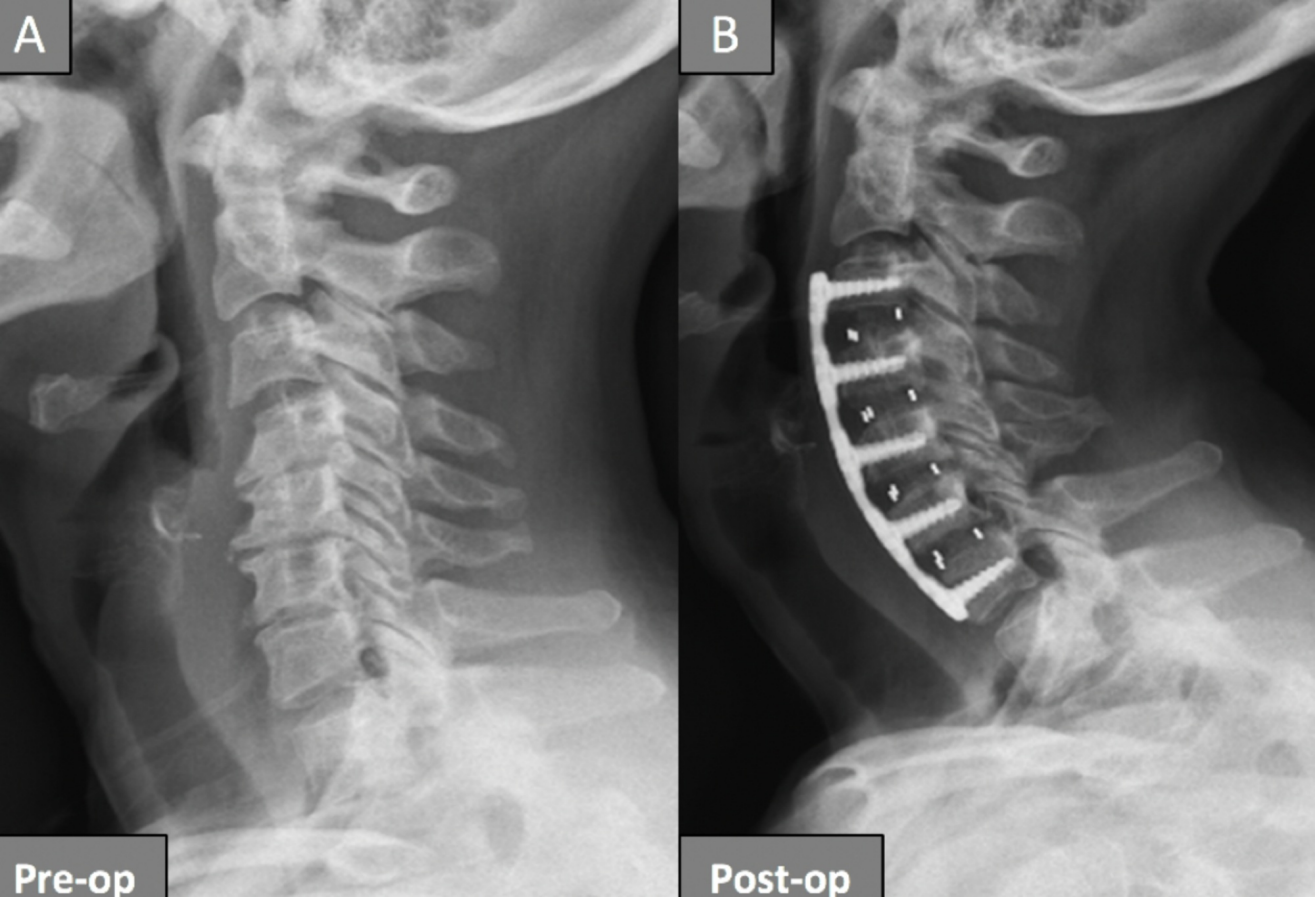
Pre- and post-operative plain films of a patient who underwent a four-level anterior cervical discectomy and fusion for kyphosis correction Plain films of a patient before surgery (A) showing loss of cervical lordosis with development of kyphosis. The same patient patient is shown after undergoing a four-level anterior cervical discectomy and fusion with kyphosis correction, showing good cervical lordosis (B). Pre-op, pre operative; Post-op, post-operative.
